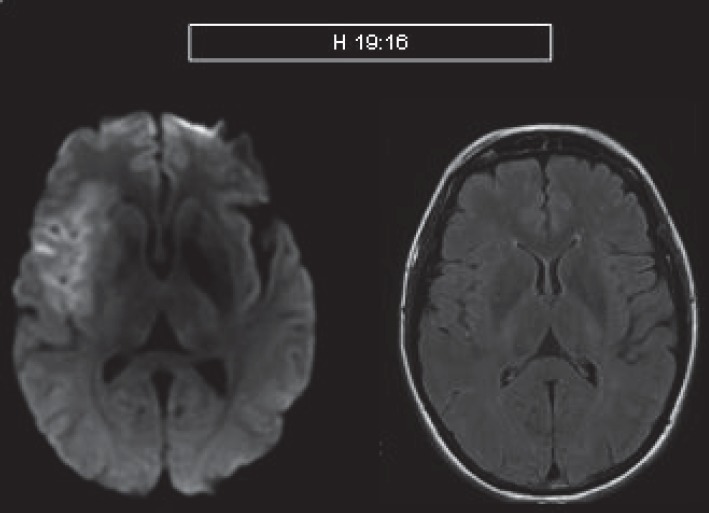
MRI diffusion with hyperintensity(left) – overacute ischemic lesion versus normal FLAIR sequence (right).
